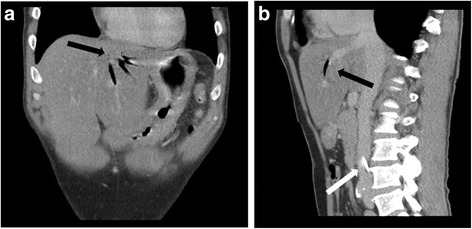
Contrast-enhanced CT scan of the abdomen several hours after a car crash with suspected blunt abdominal trauma showing gas formation within the left liver vein (black arrow a, b). Furthermore, the central venous catheter is seen in the vena cava inferior (white arrow, b)
